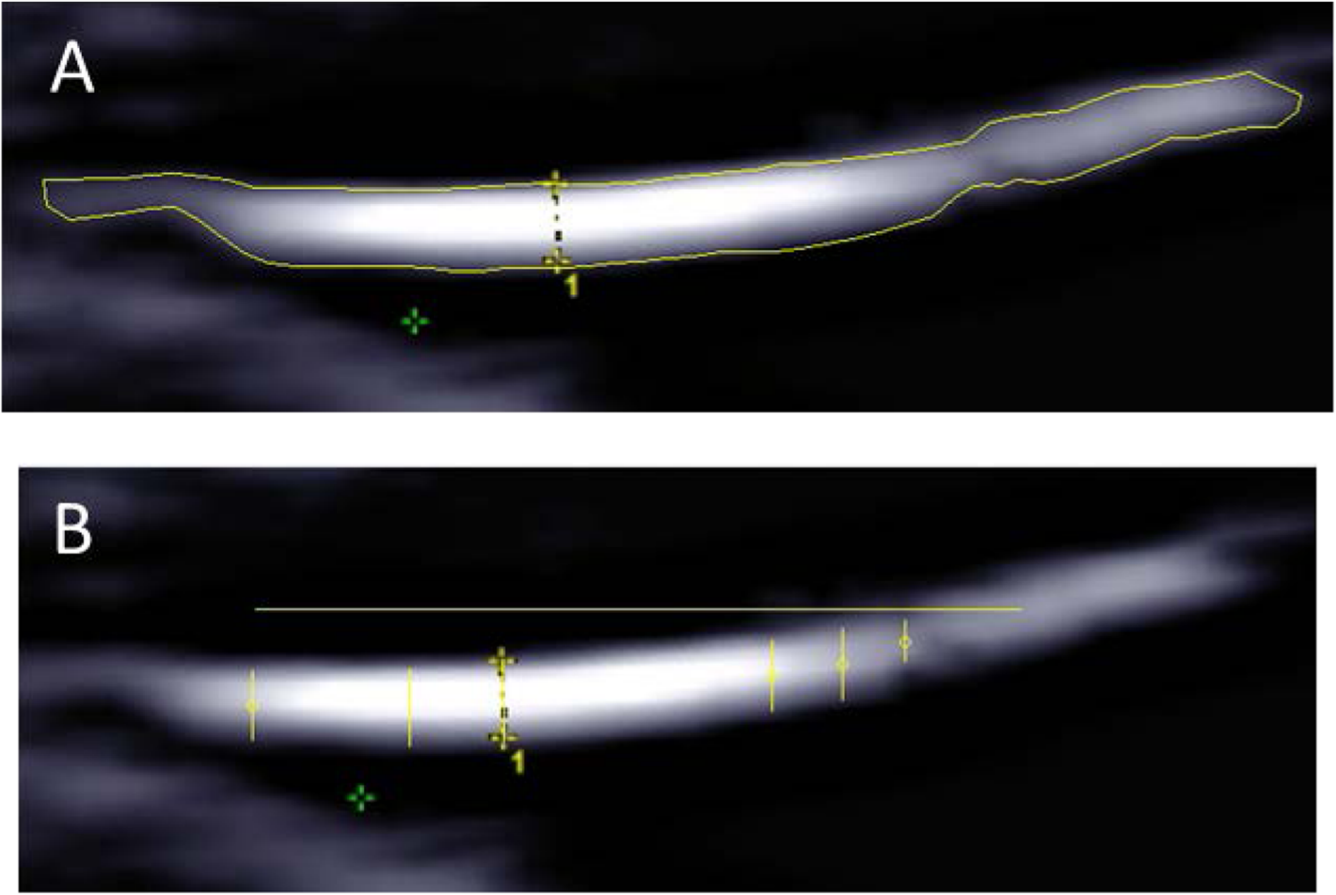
Ultrasound image of fetal membranes at 21 weeks 4 days, obtained transvaginally. a An outline was manually drawn around the membranes to determine the area and length, which were used to approximate the average thickness using the equation: average thickness = area/length. b Six thickness measurements were performed along a 1 cm range near the center of the image of the membranes (1 cm scale bar along the top) to identify the maximum and minimum thicknesses. Values are provided in Table 3
